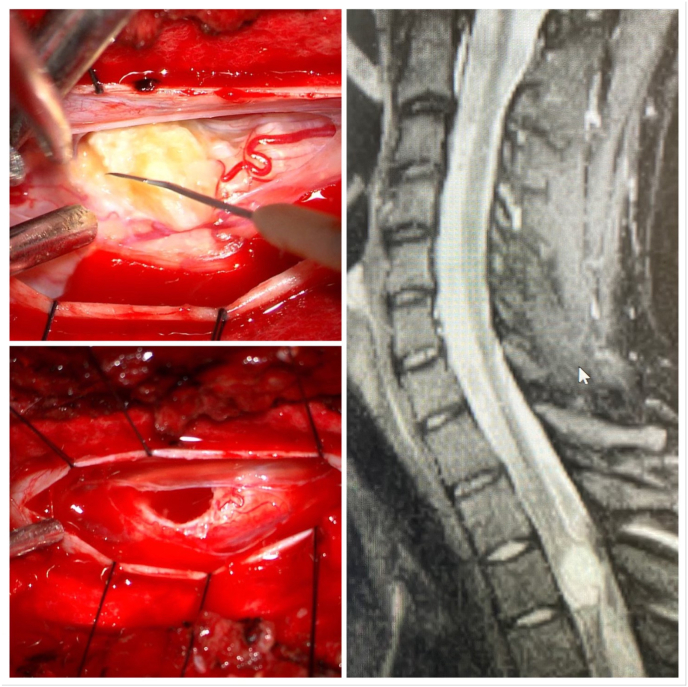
Intraoperative photographs showing an exophitic, yellowish, pearly tumor emanating from the right side of spinal cord at T3 (Left, Above). The cavity of the dermoid cyst after complete resection (Left, Bottom). Sagittal T1-weighted image revealed a well-encapsulated hypointense lesion within the spinal canal resulting in cord compression (Right).
